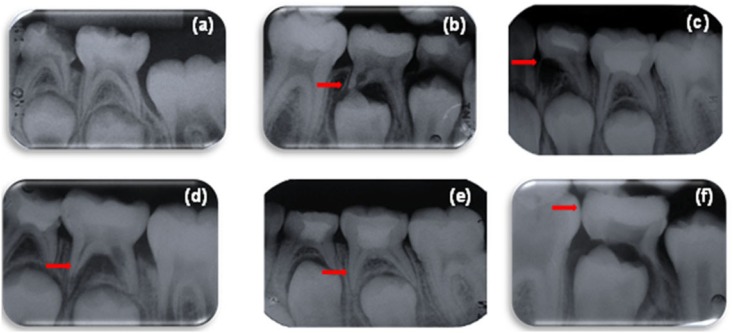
Sample radiographs representative of radiographic changes during follow-up. Arrows indicate the region of interest. (a) Preoperative radiograph; (b) Internal root resorption without perforation; (c) Internal root resorption with perforation; (d) Inter-radicular bone destructions; (e) Radiographic success; (f) Tooth too close to exfoliation
